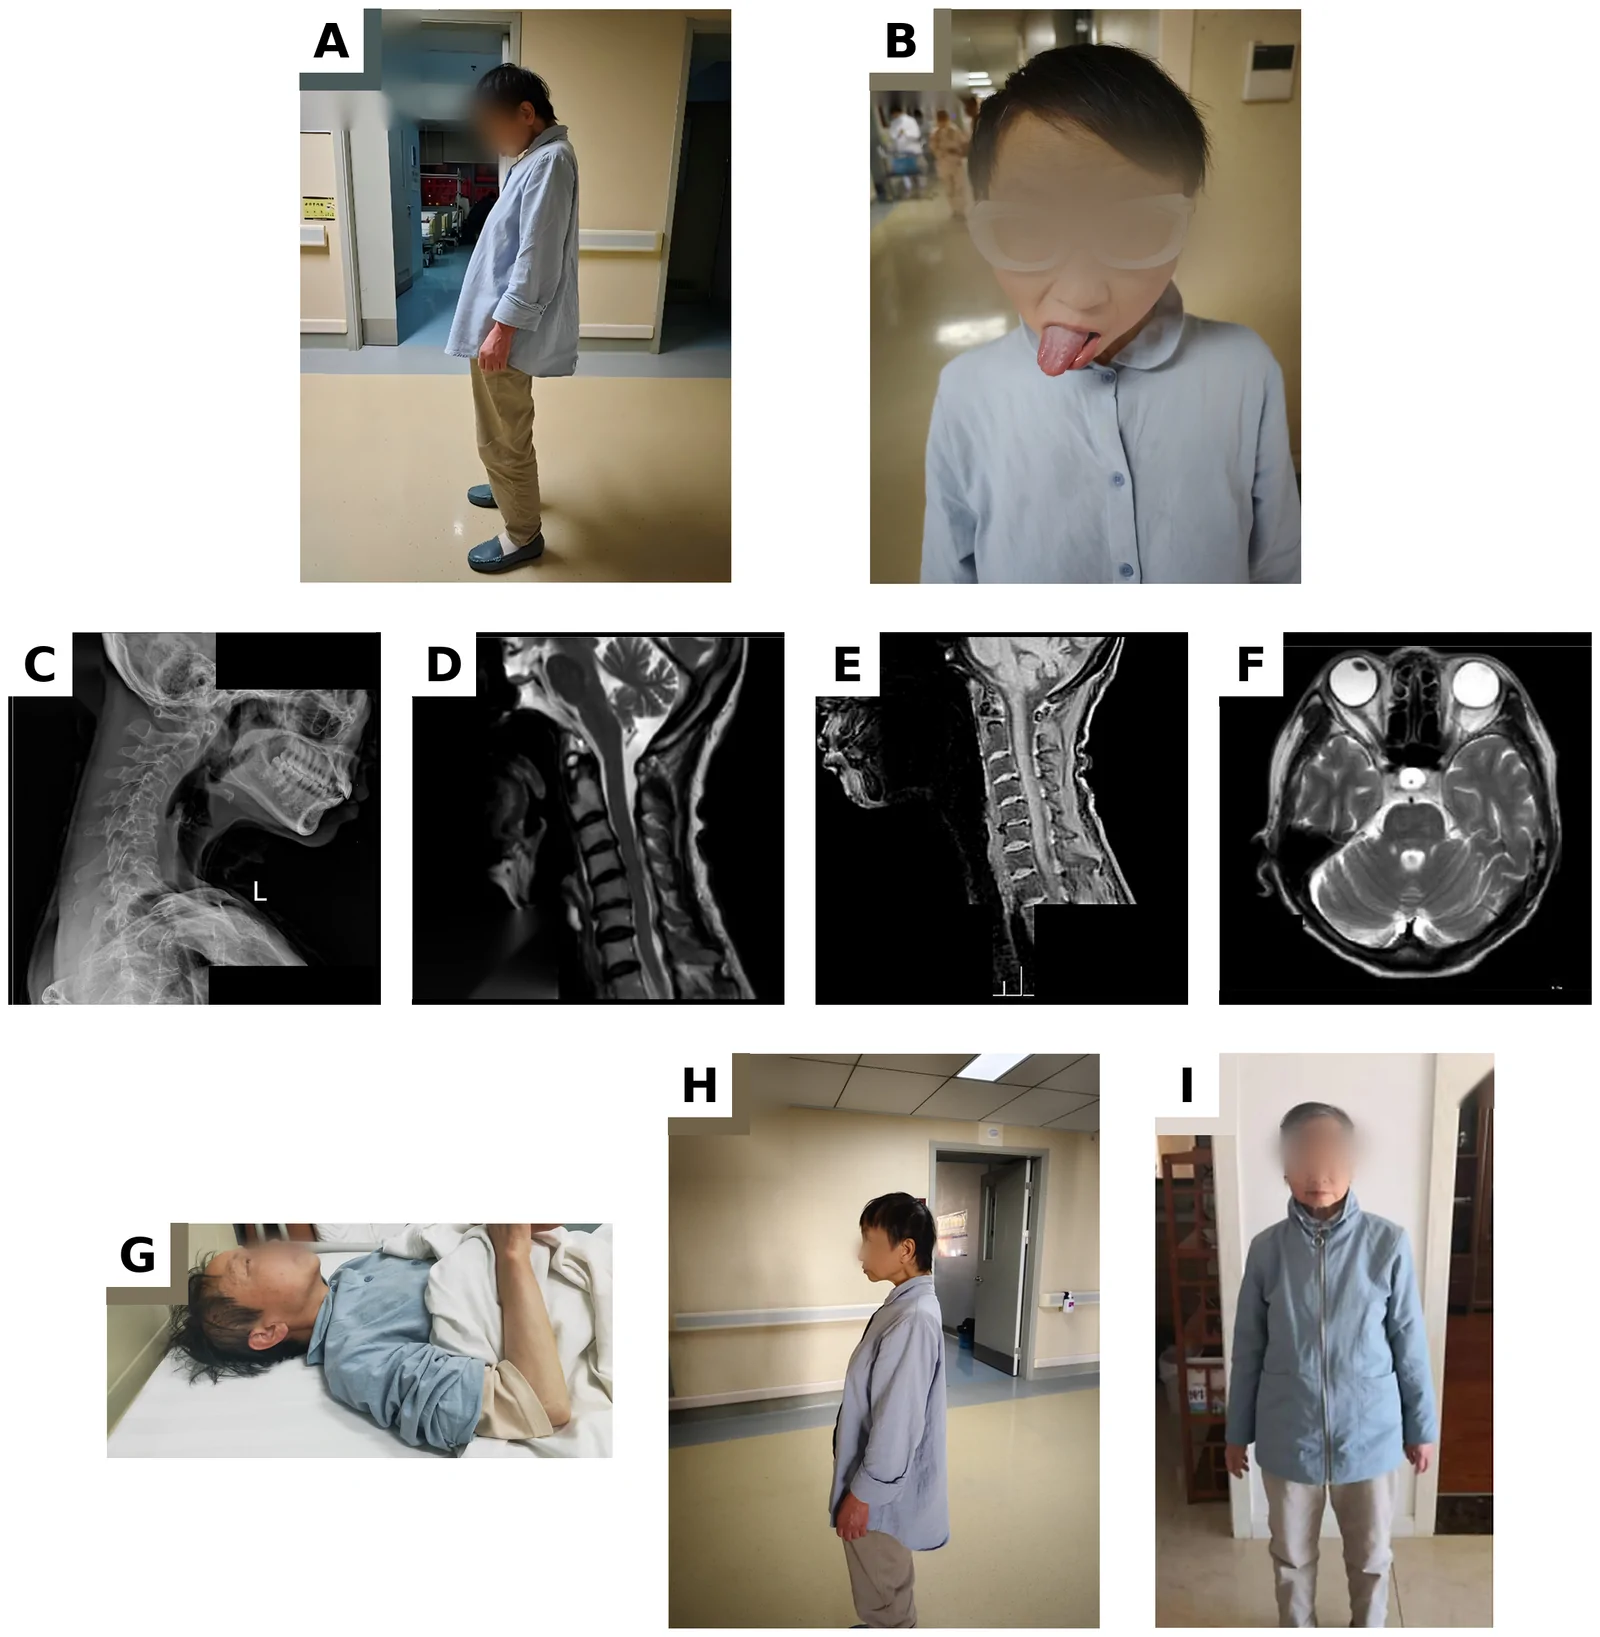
Clinical course from admission to one-year follow-up. (A) Admission: severe chin-on-chest deformity on standing. (B) Admission: rightward deviation of the tongue on protrusion, consistent with left hypoglossal-nucleus dysfunction. (C) Cervical radiograph (lateral): kyphosis with C2–C3 anterior translation and multilevel degenerative change. (D) T2-weighted sagittal cervical MRI: multilevel disc protrusion, C3–C7 canal stenosis with mild cord indentation. (E) Contrast-enhanced (gadobutrol) T1-weighted sagittal image of the craniocervical junction: no abnormal enhancement of the lower brainstem or cervical cord. (F) T2-weighted axial brain MRI at the level of the pons: no acute brainstem parenchymal signal abnormality. (G) Hospital day 9: able to lie supine without pillow support, indicating the earliest recovery of tonic neck-extensor activity. (H) Hospital day 11: erect standing posture with full active cervical extension [compare with (A)]. (I) One-year outpatient follow-up: complete resolution of cervical kyphosis with sustained upright posture and no recurrent deformity. Scale bars are not applicable for clinical photography.
